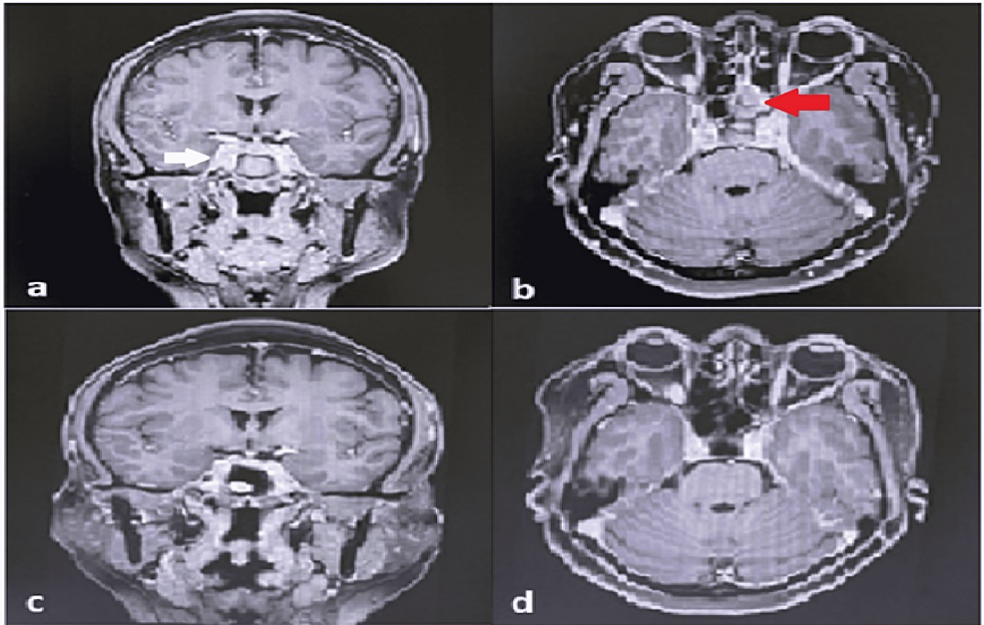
Plain and contrast-enhanced cranial magnetic resonance imaging of the patient (a) T1 post-contrast coronal cut shows prominent abnormal enhancement involving the cavernous sinuses accompanied by interspersed internal non-enhancing abnormalities with an asymmetric degree of involvement of the right cavernous sinus (white arrow); (b) T1 post-contrast shows the inspissated secretions within the left sphenoid sinus. (red arrow); (c) T1 post contrast coronal cut. A follow-up study showed no abnormality involving the cavernous sinuses. The cavernous sinuses demonstrate normal flow and contrast enhancement; (d) The T1 post-contrast follow-up study demonstrates the absence of left sphenoid sinus secretions.
